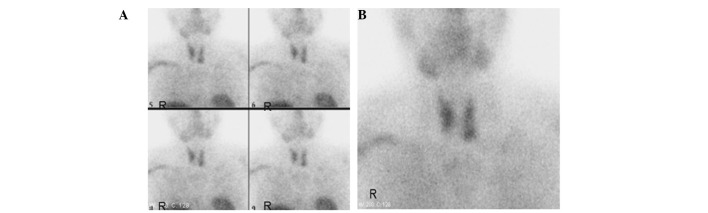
Parathyroid emission computed tomography. (A) Blood pool images and (B) a 15 min static image of the neck.
